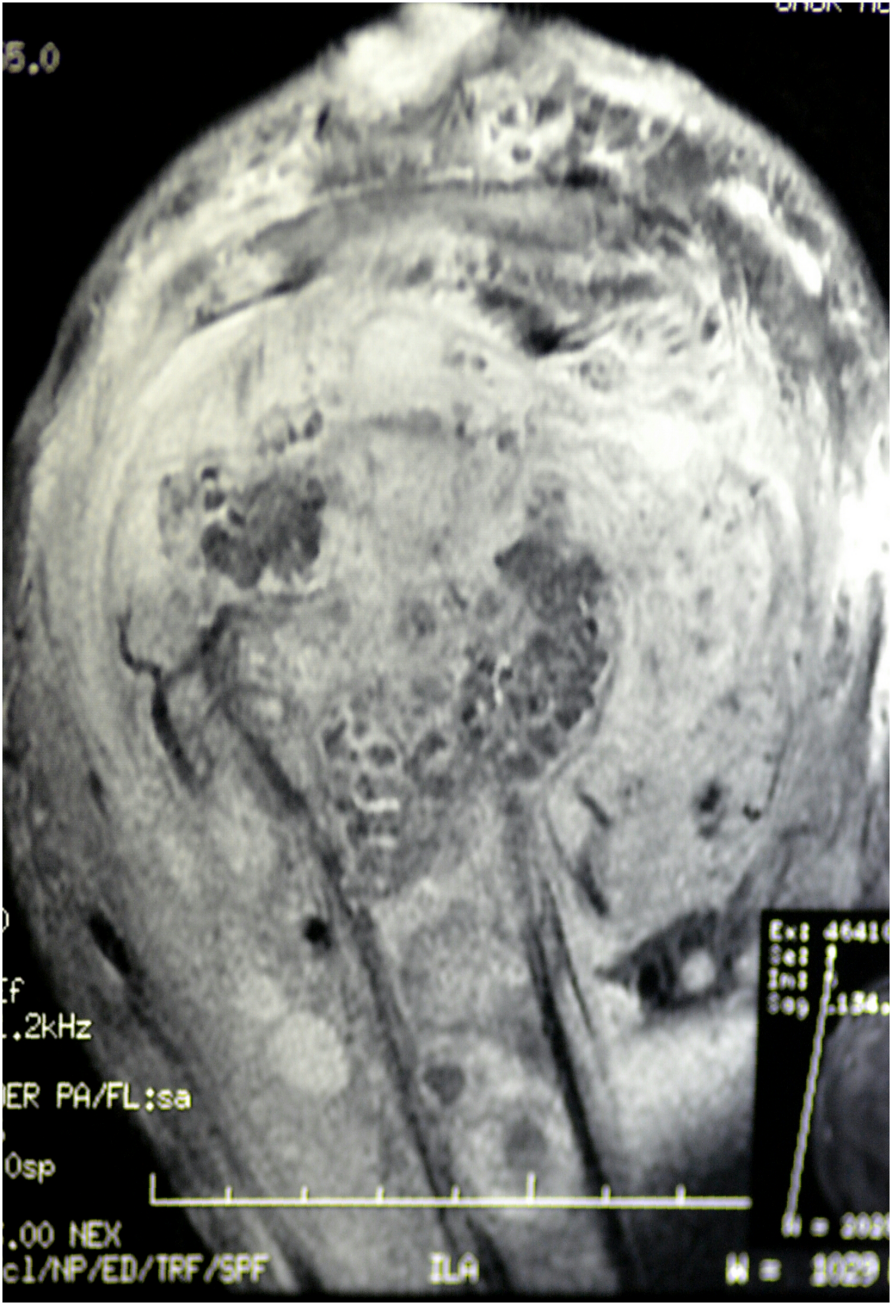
MRI showing innumerable soft tissue lesions infiltrating the rotator cuff muscles with extension into the humeral head, proximal humeral shaft, and glenoid aspect of the scapula. The acromioclavicular and glenohumeral joints were also affected, causing widening of the joint spaces. Multiple collections within affected bones and subcutaneous soft tissues consistent with abscess formation were seen. Joint effusion was noted, along with enlarged left axillary lymph nodes.
